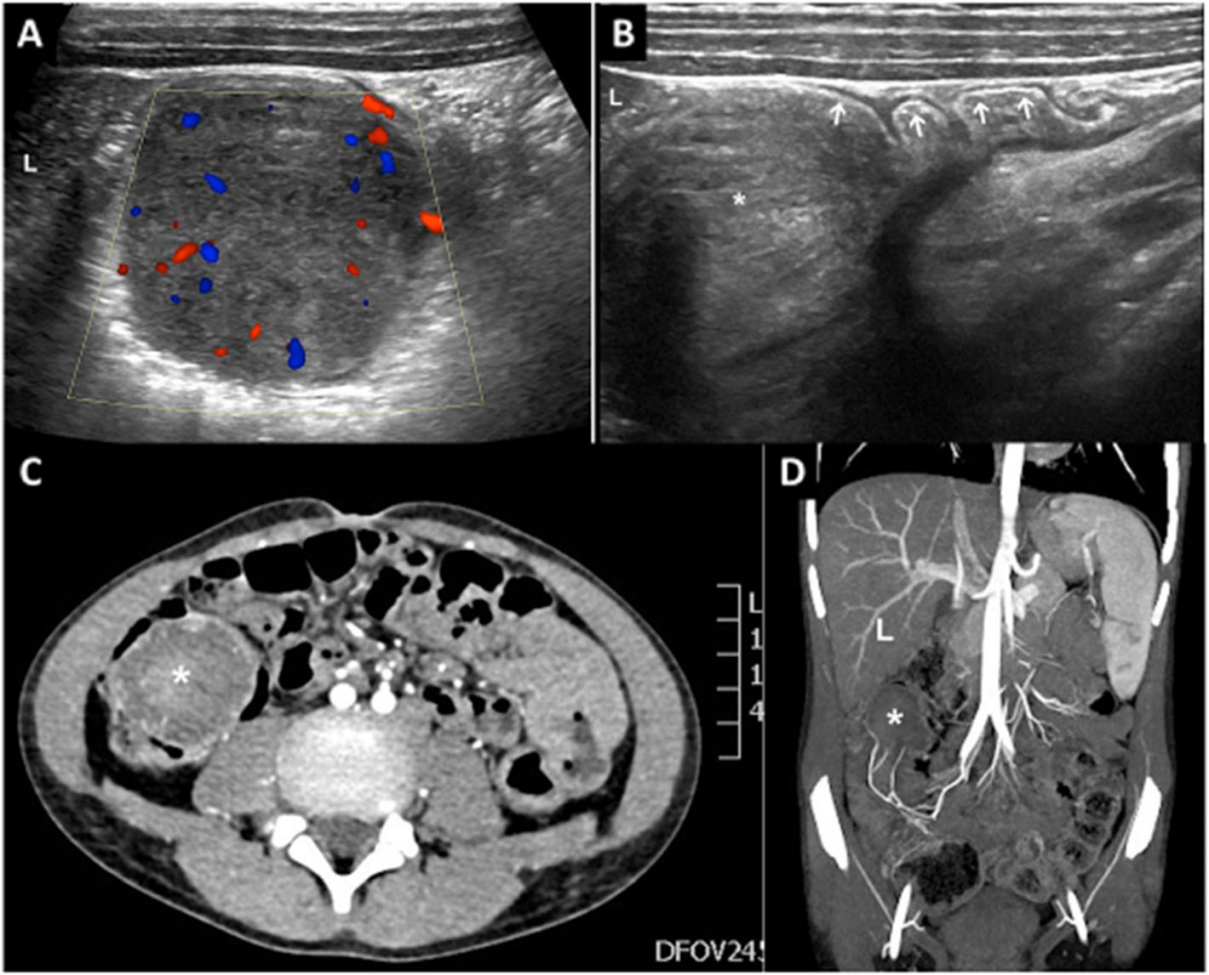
US Axial scan (a) shows a solid rounding mass in subheptical seat with intralesional vascularization; US longitudinal scan (b) shows a rounded mass (*) in the lumen of the colon (arrows); CT contrast exam, axial (c) and coronal (d) scan, show rounding solid mass (*) in subhepatic site. L = Liver
